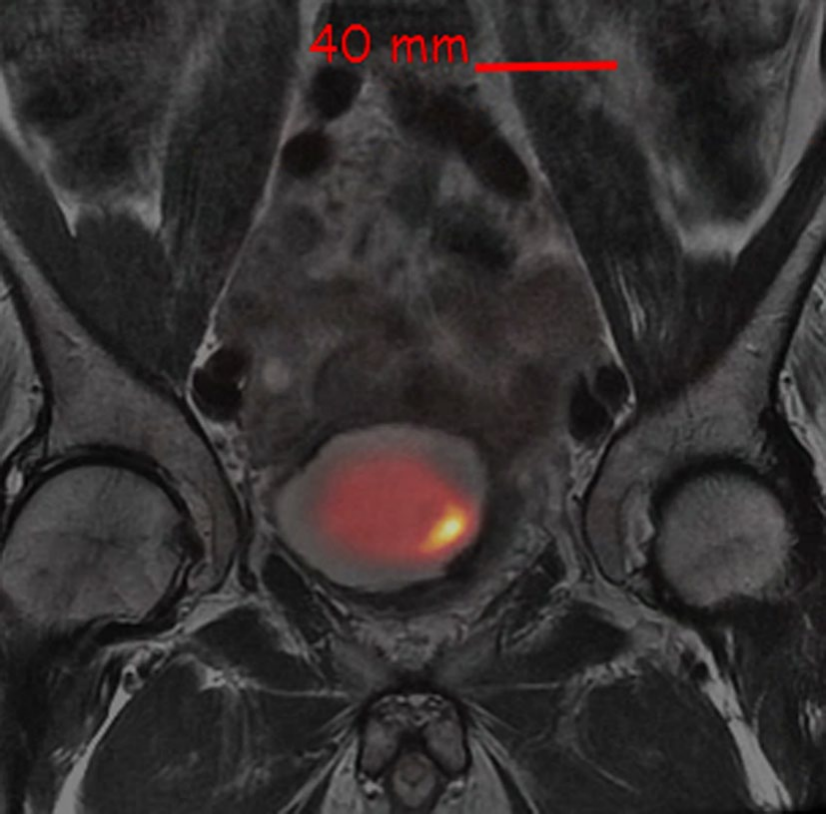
Fused PET and MRI, using bone as the landmark
